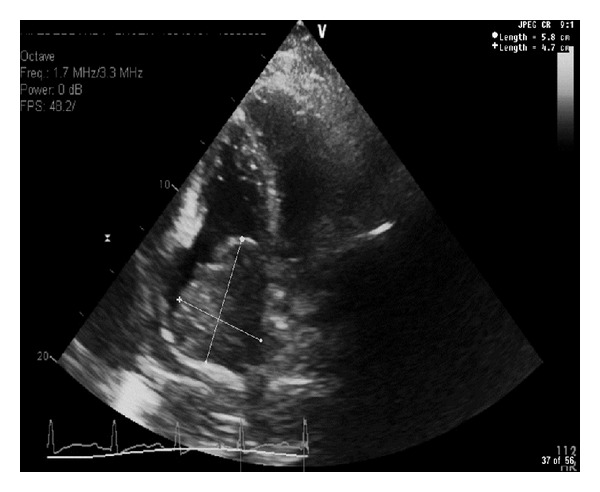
A comprehensive 2 dimensional M-mode color flow and Doppler echocardiography reveals a normal left ventricular systolic function (EF 60–69%). A large right atrial mass measuring 5.8 × 4.7 cm almost fills the right atrium and extends into the tricuspid valve causing tricuspid regurgitation.
